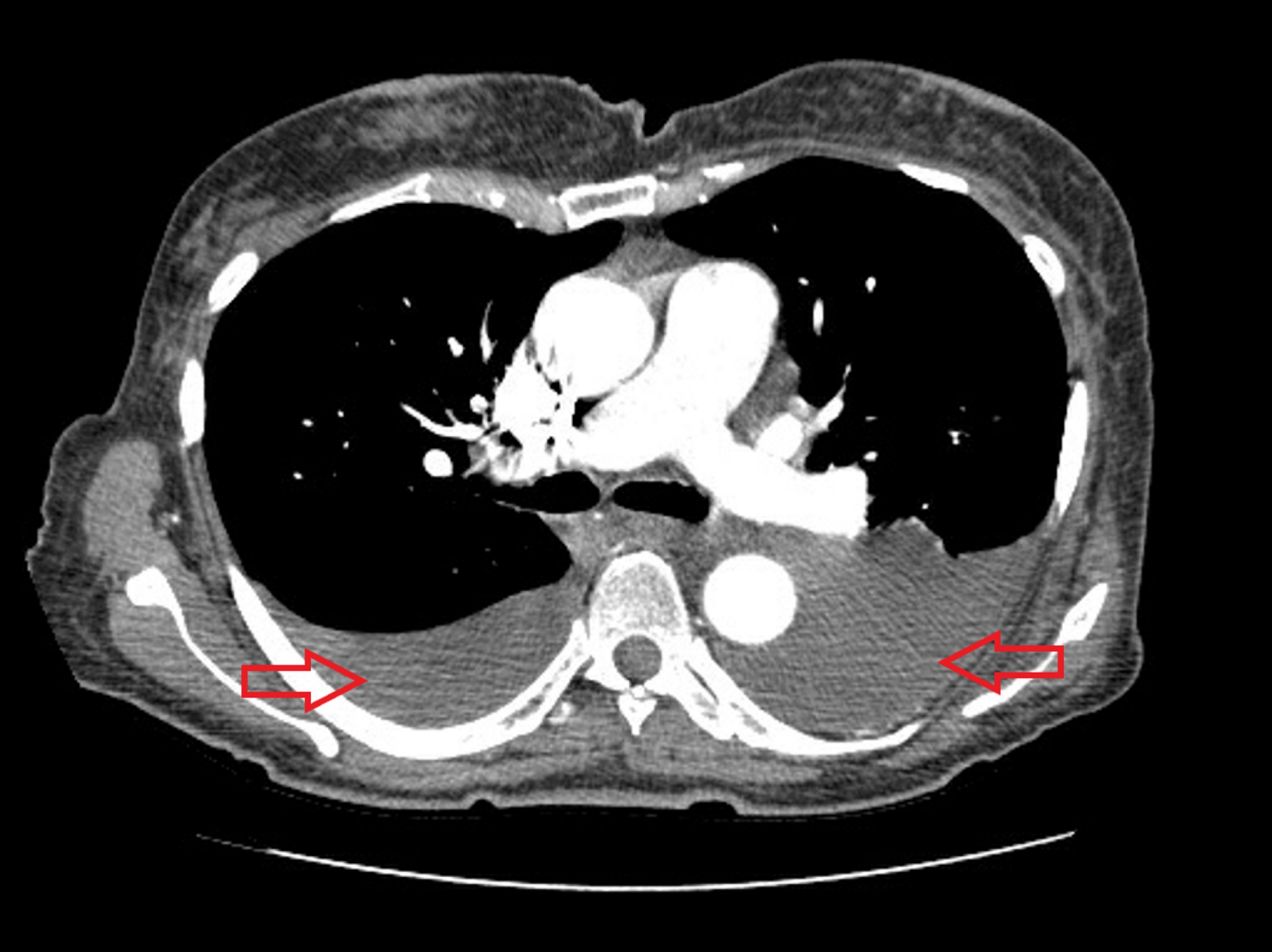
CTA performed on previous admission (April 20, 2020) showing no evidence of pulmonary embolism but bilateral pleural effusion (red arrows) CTA, computed tomography angiogram
